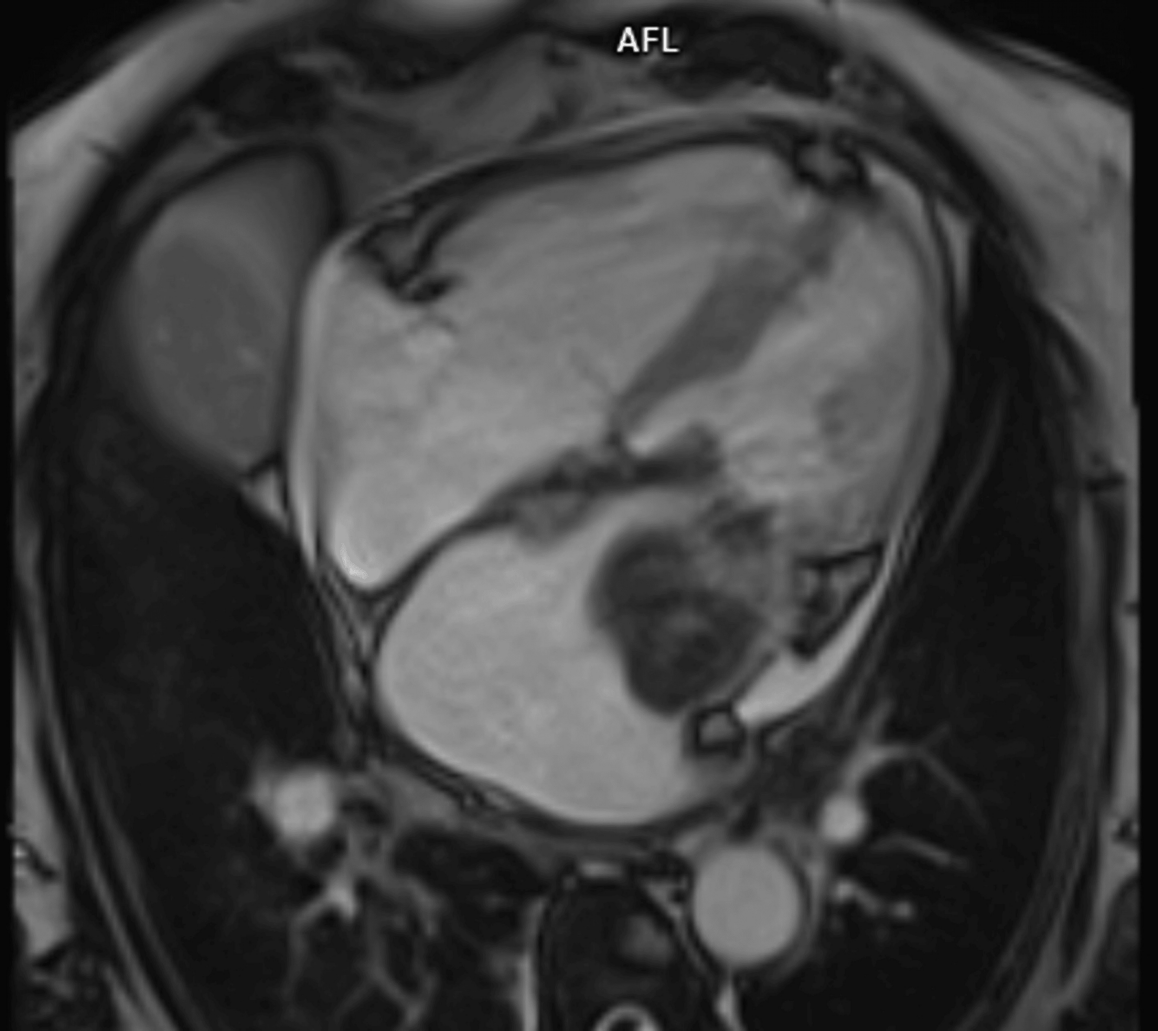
Cardiac magnetic resonance imaging showing the real-time dynamic four-chamber view
